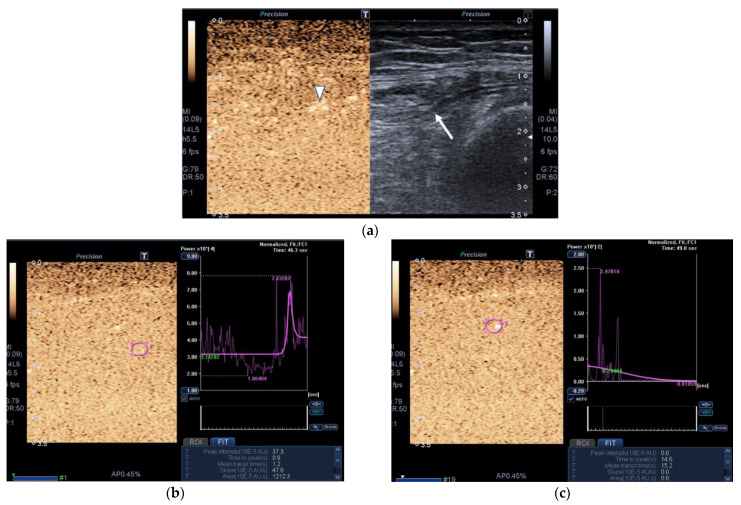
Transversal QRF muscle of a patient with multiorgan failure (77 years old). (a). Transversal ultrasonography scan shows the QRF muscle (arrow) and vascularization after contrast-enhanced ultrasound (CEUS) administration (arrowhead). CEUS imaging and time–intensity curve analysis are shown using the built-in software. (b) ROI (pink circle) in the area of strongest enhancement indicates maximum perfusion in the QRF. (c) ROI (pink circle) in the area of lowest enhancement indicates minimum perfusion in the QRF.
